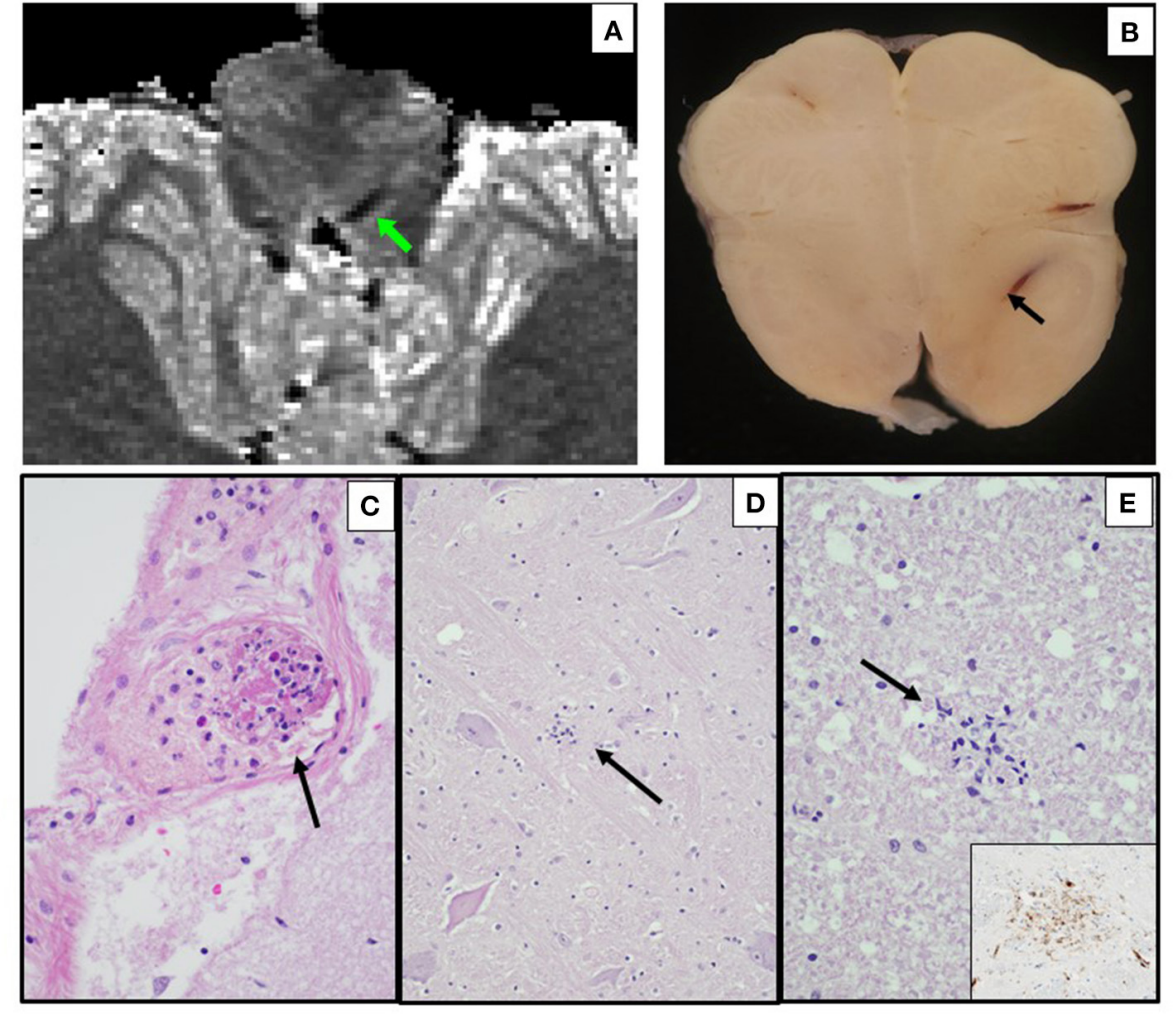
Ex vivo* MRI and autopsy neuropathology in Case 6. (A)
